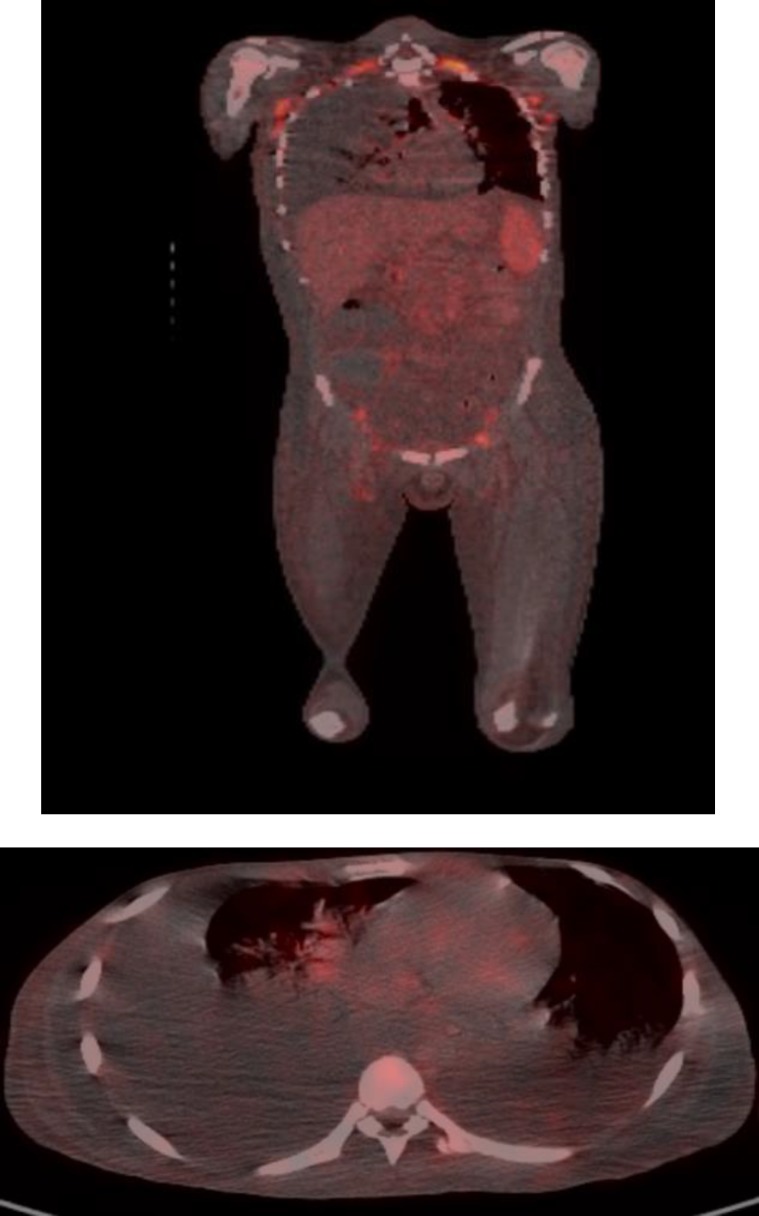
Multiple lymphadenopathies in cervical, axillary, mediastinal, paraaortic, mesenteric and inguinal lymph nodes. FDG uptake rates were from 3.6 to 8.5.
